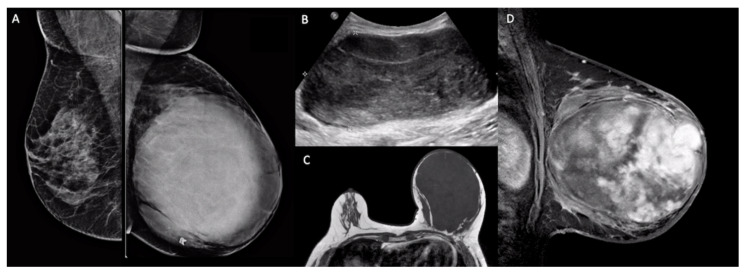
Imaging findings of a malignant phyllodes tumor. (A): Bilateral mammography (mediolateral oblique view) showed a large, round, circumscribed, high-density mass in the left breast. (B): Breast ultrasound showed an oval, parallel, circumscribed mass with a heterogeneous echo pattern. (C): T1-weighted axial magnetic resonance imaging (MRI) showed a large, hypointense, circumscribed mass in the left breast. (D): T1-weighted sagittal fat-saturated contrast-enhanced MRI showed heterogeneous internal enhancement within the mass.
